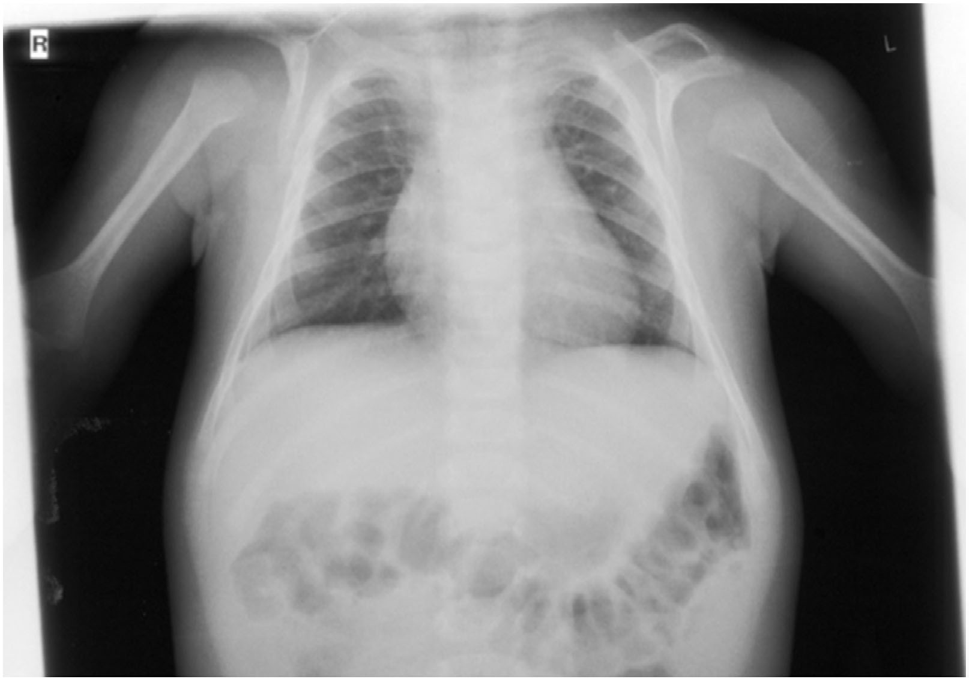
X-ray (detail of a babygram) of the patient at the age of 7 months showing a hook-shaped hypoplastic left clavicle.
